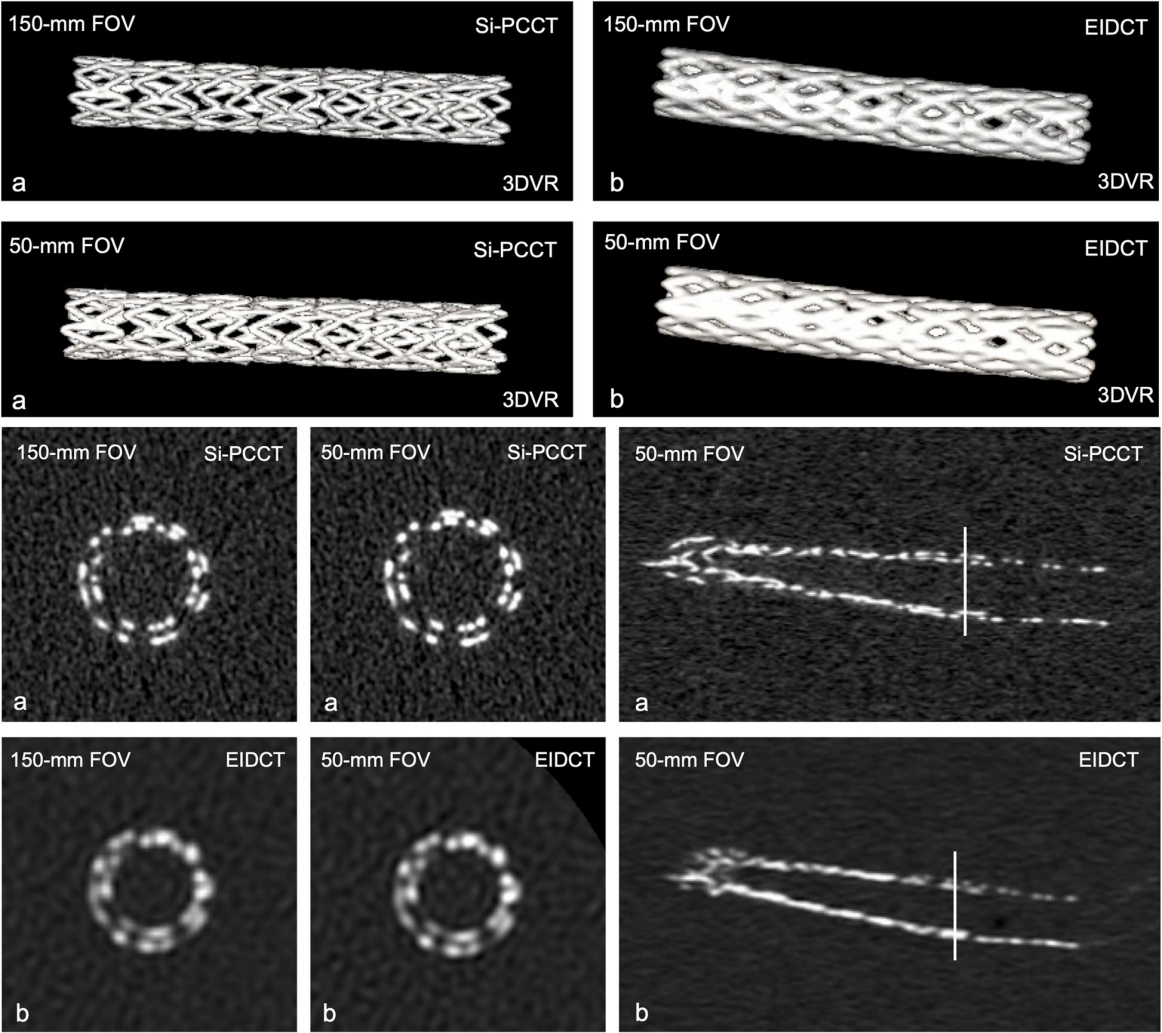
Three-dimensional volume-rendered (3DVR) images, axial and coronal slices at 150-mm and 50-mm field-of-view (FOV) for silicon-based photon-counting CT (Si-PCCT) (a) and energy-integrating detector CT (EIDCT) (b). Qualitative stent appearance was found to be insufficient (score 1) for 12.5% of the EIDCT images (both FOVs) due to impaired visibility of stent struts and impaired stent delineation, as represented in the 3DVR images
